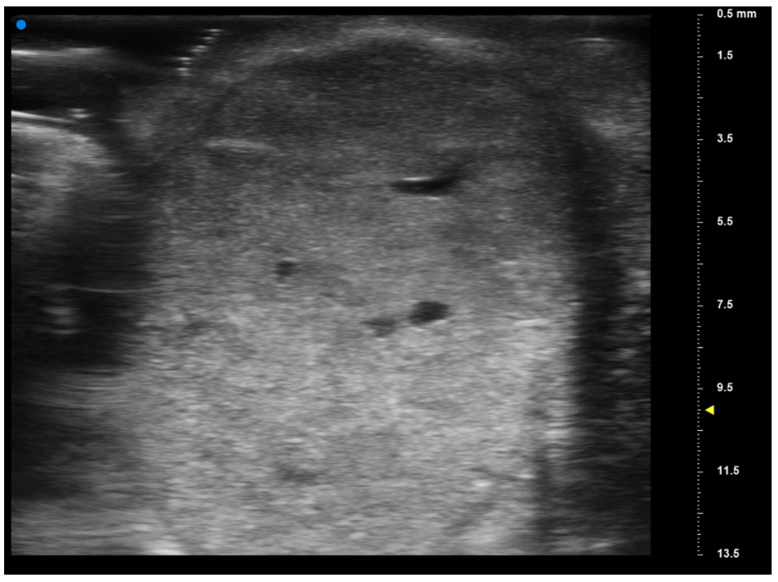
The high-frequency ultrasound depicts a well-defined round-shaped inhomogeneous hyperechoic lesion within the oral mucosa consistent with a mucoepidermoid carcinoma. The lesion exhibits regular borders and is confined to the deeper layers of the mucosa. There are heterogeneous areas within the lesion, reflecting mixed echogenicity, suggestive of varying tissue compositions. Surrounding tissues do not show any apparent signs of invasion.
